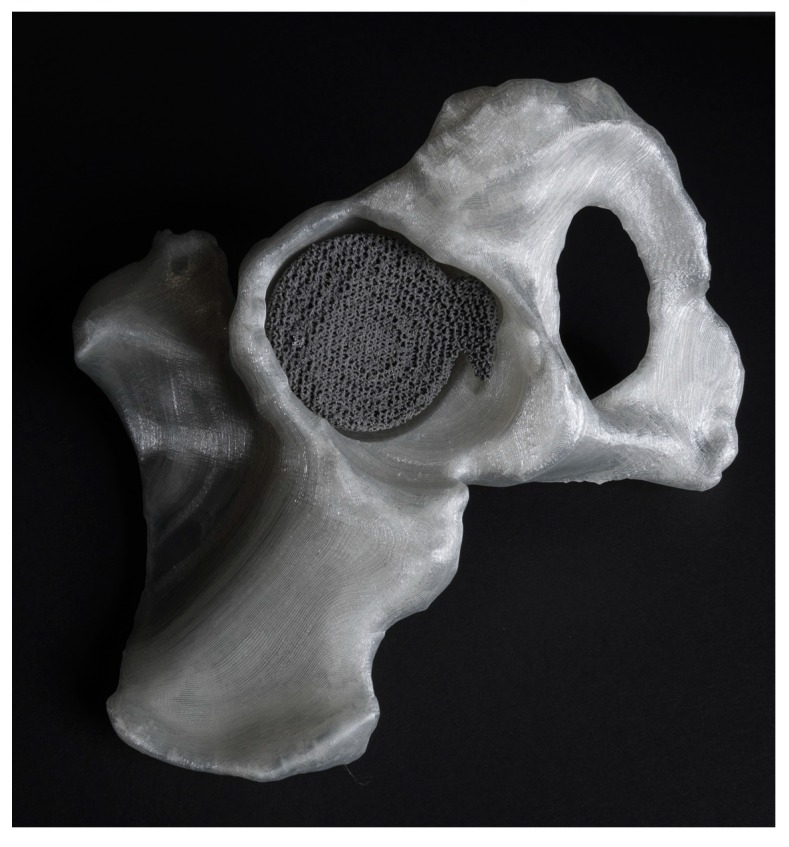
A patient-specific implant designed based on computed-tomography (CT) images and AM with selective laser melting. The corresponding bone is also segmented from CT images and AM using with fused deposition modeling. Made at Additive Manufacturing Lab, TU Delft (Researchers: Eline Kolken and Saber Amin Yavari).
